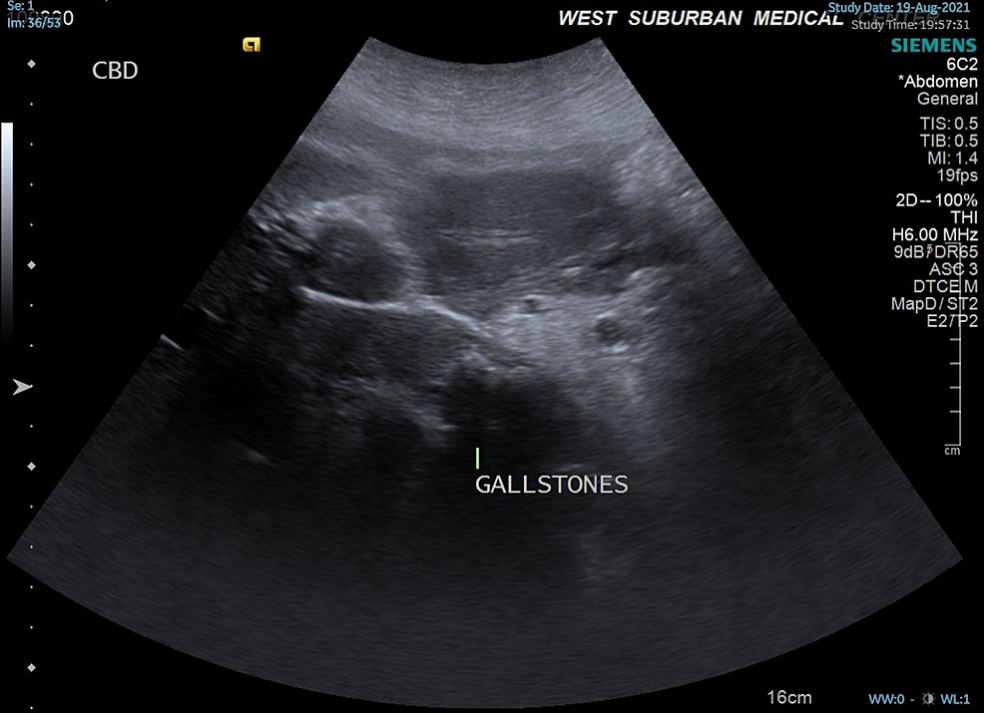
Ultrasound image showing multiple gallstones.
